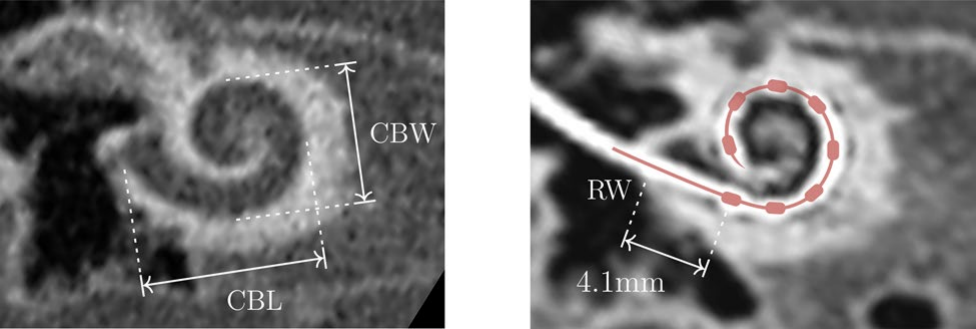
Example of a preoperative (left) and a postoperative (right) CT scan. From the preoperative image, the cochlear base length (CBL) and width (CBW) can be estimated in millimeter. After the implantation, the insertion depth of the electrode array can be assessed by measuring the distance between the round window (RW) and the most basal electrode. In this example, the insertion depth corresponds to 4.1 mm. For visual simplicity, only the eight most basal electrodes are annotated in the postoperative scan
